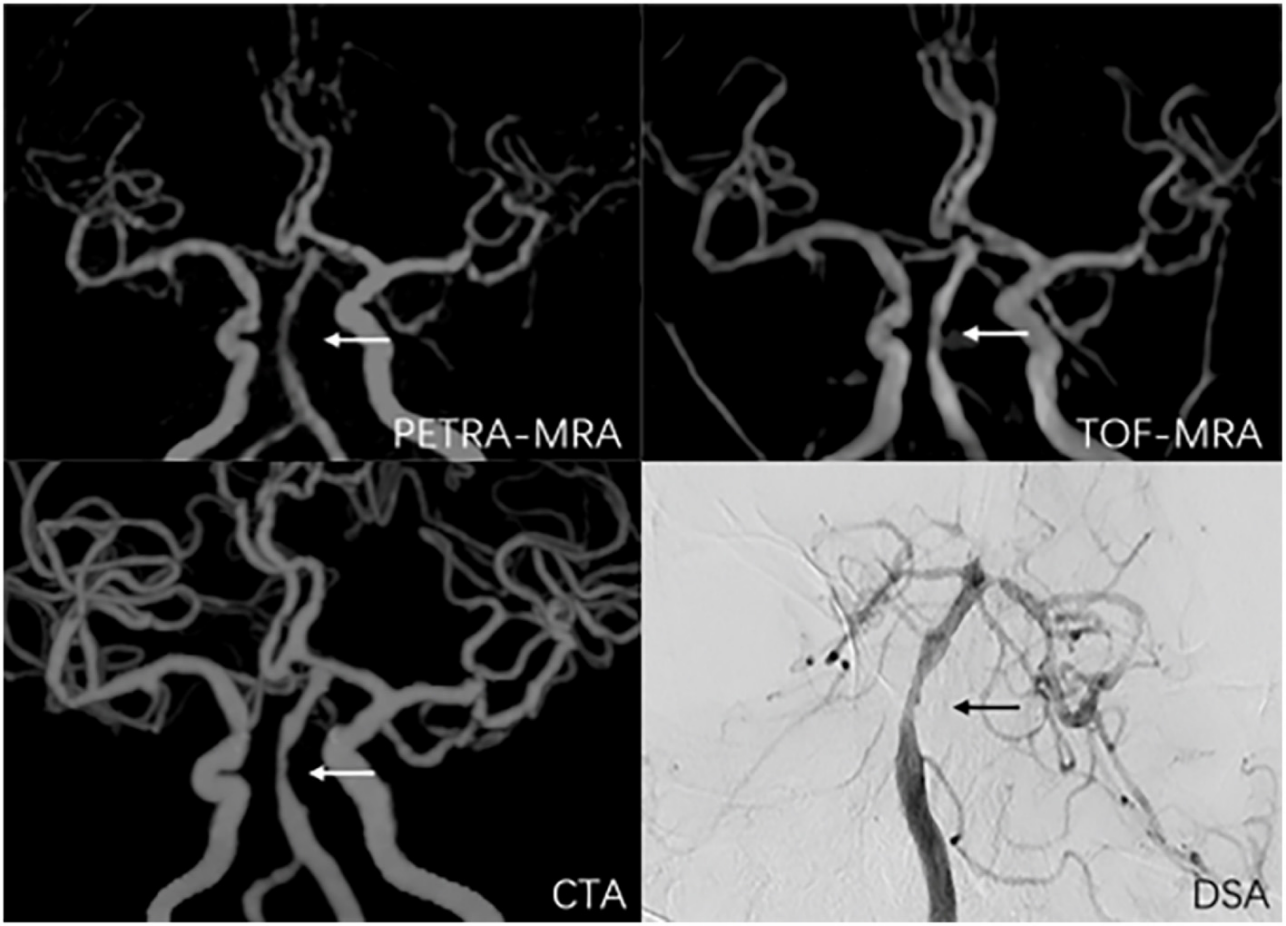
A 56-year-old man with symptomatic basilar artery stenosis. The arrow marks the location of the lesion. The degree of stenosis was 59.9% on PETRA-MRA, 51.1% on TOF-MRA, 69.7% on CTA, and 50.3% on DSA. Lesion length was 5.36 mm on PETRA-MRA, 5.43 mm on TOF-MRA, 5.76 mm on CTA, and 5.05 mm on DSA.
